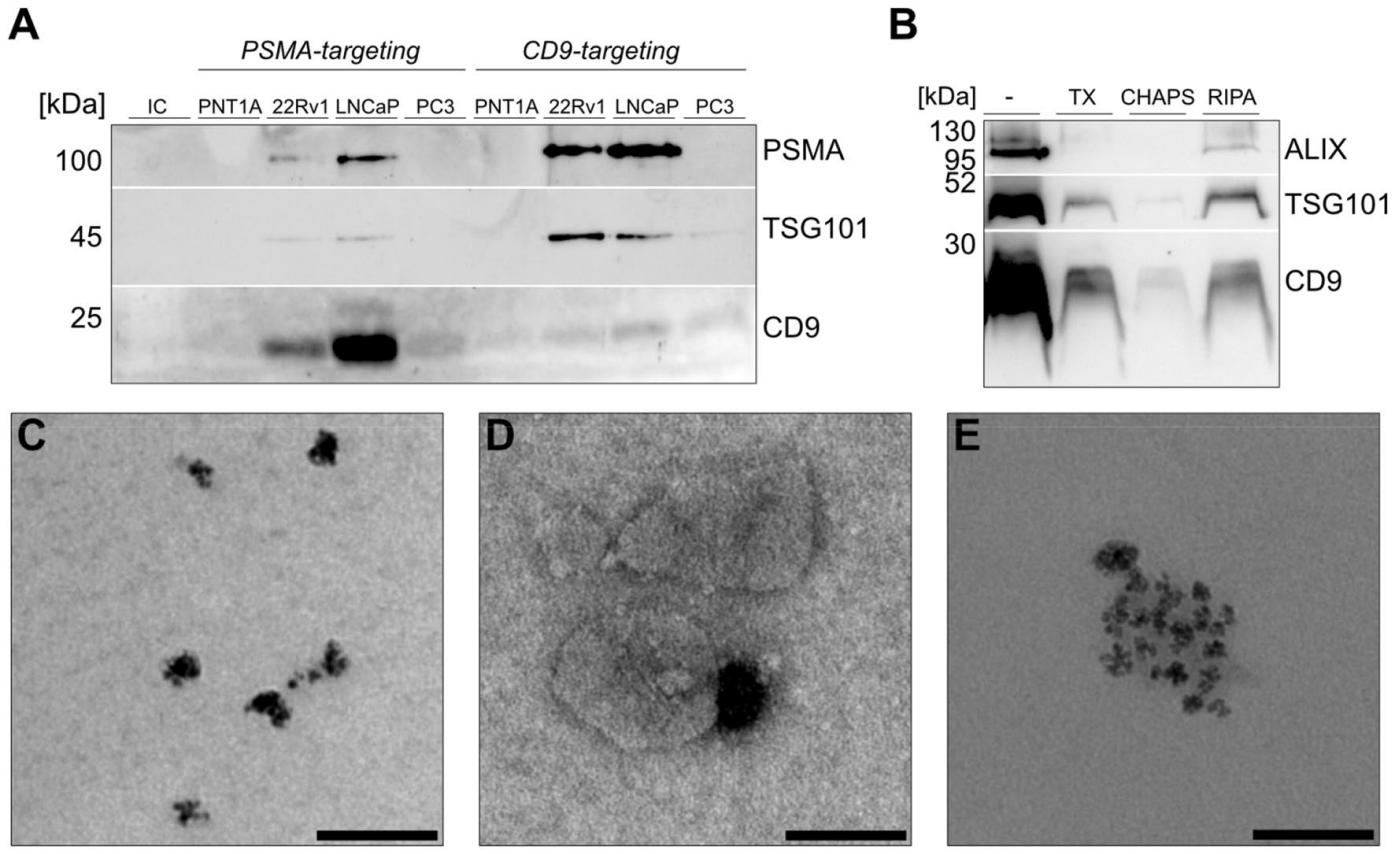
Immunomagnetic bead isolation of EVs targeting PSMA or CD9 from PNT1A, 22Rv1, LNCaP and PC3 ultrafiltrated supernatant after 24 or 30 h serum-free incubation of 2E+10 particles compared to the isotype control (IC) (A) and lysis of CD9-targeted EVs from 22Rv1 UF after Triton X-100, CHAPS and RIPA compared to the untreated control (−) by western blot (B) TEM of Streptavidin MicroBeads (C) and CD9-targeted EVs from 22Rv1 of 50 k fold magnification (D, E). Scale bars represent 100 nm (n = 2).
